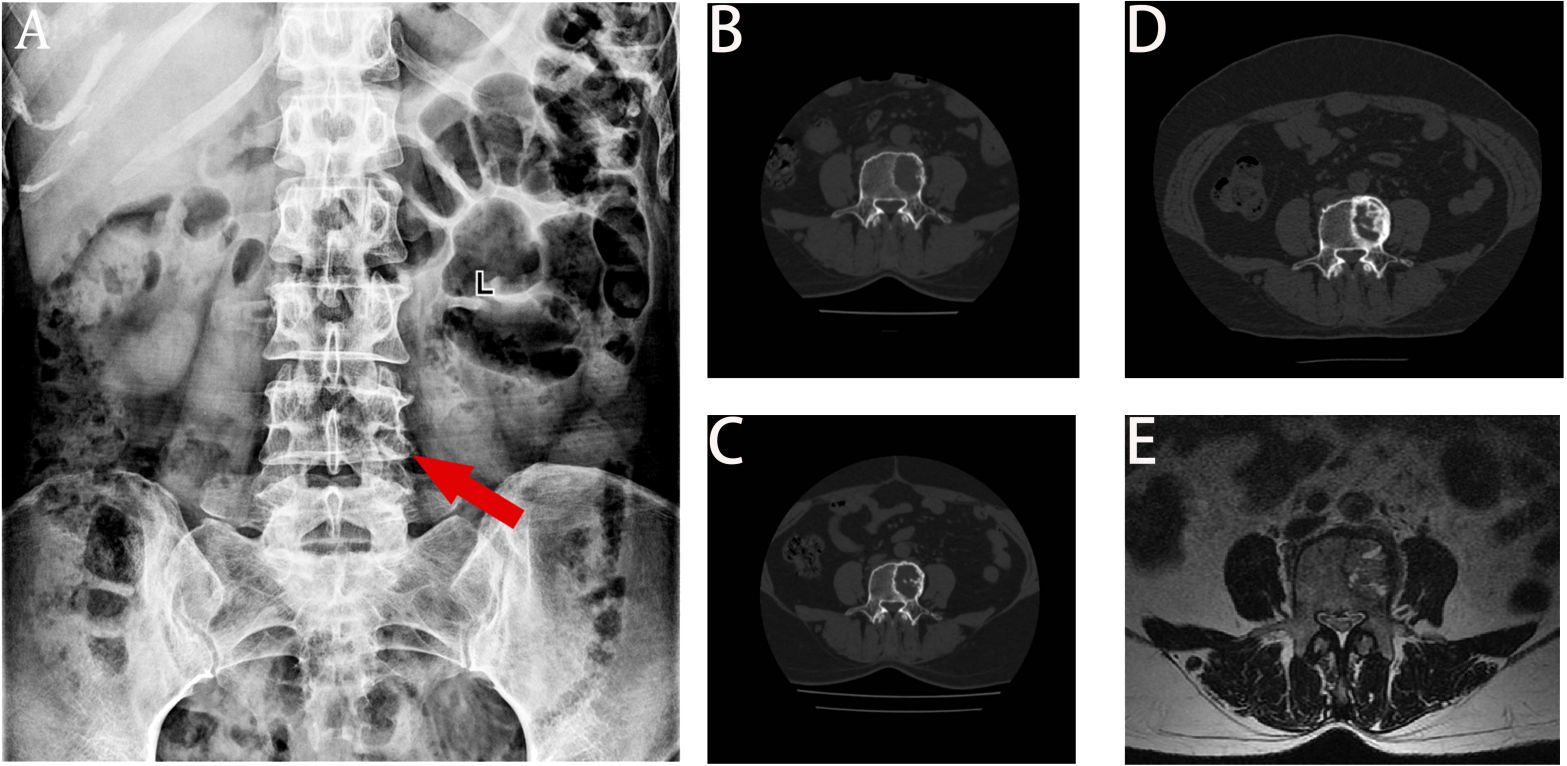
X-ray, CT, and MRI images. Panel (A) displays the flattening of the L4 left vertebral body height. Panel (B) displays the bone destruction of L4 before chemotherapy, with a moderately circular low-density shadow with a range of 3.4 cm × 2.0 cm × 2.2 cm. Panel (C) displays a decrease in lytic bone destruction and an increase in the cortical bone density in posterior L4 after six cycles of chemotherapy. Panel (D) displays obvious calcification in the bone destruction area of the L4 vertebra at the last review. Panel (E) displays that the left side of the L4 vertebra is slightly flattened, with patchy long T1 equal length T2 signals and high T2 pressure lipid images.
